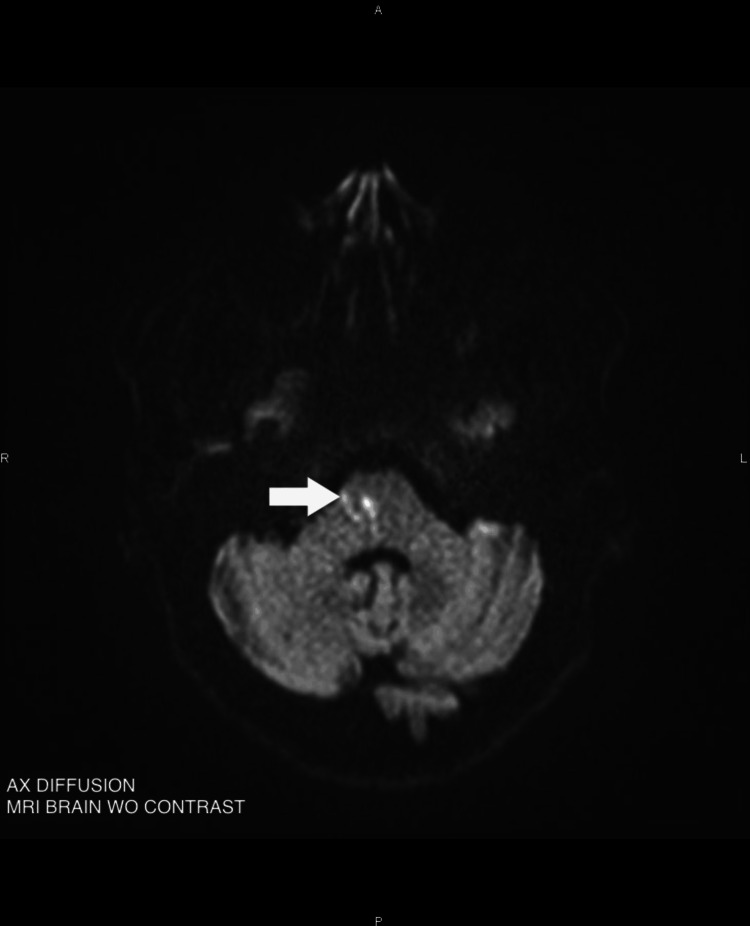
Brain MRI White arrow: Hyperintense lesion on diffusion sequence in the right inferolateral pons; AX: Axial; WO: Without
